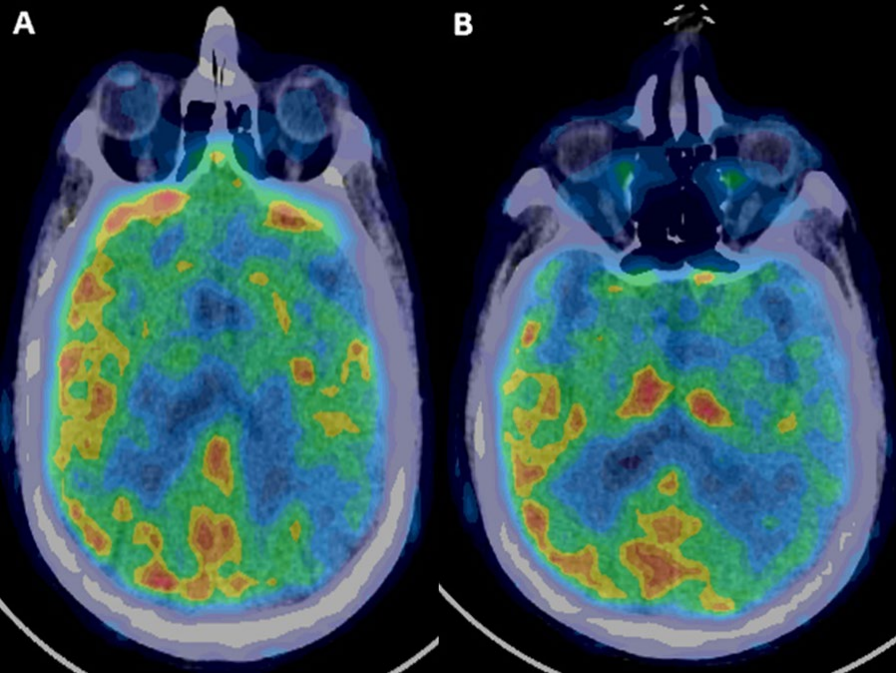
Positron emission tomography (PET) with ^18^fluorodeoxyglucose shows hypometabolism in left temporal lobe and adjacent parietal and occipital lobes on ^18^fluorodeoxyglucose positron emission tomography (FDG PET)
